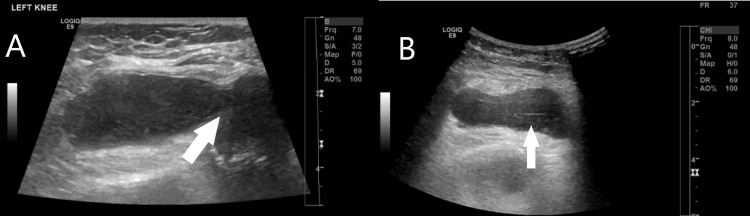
Ultrasound of the left knee showing different cuts (A and B) where the arrows point at periarticular soft tissue swelling. Distension of the suprapatellar bursa (arrow in B) is evident. Synovial thickening with minimal fluid in the knee joint (arrow in A) is also noted
